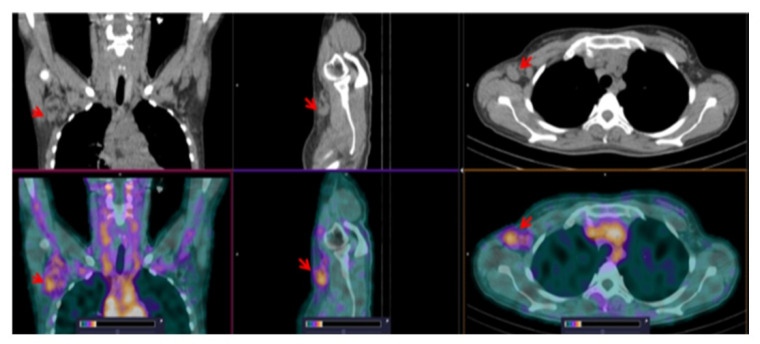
SPECT/CT images of patient 11 at 2 h after injection of [^99m^Tc]Tc-TG. An enlarged (up to 2.2 cm) right axillary node with elevated [^99m^Tc]Tc-TG uptake (SUVmax = 1.86) is visualized (arrows). The upper setting of the scale window (22% of the maximum number) was adjusted to visualize the lesion.
